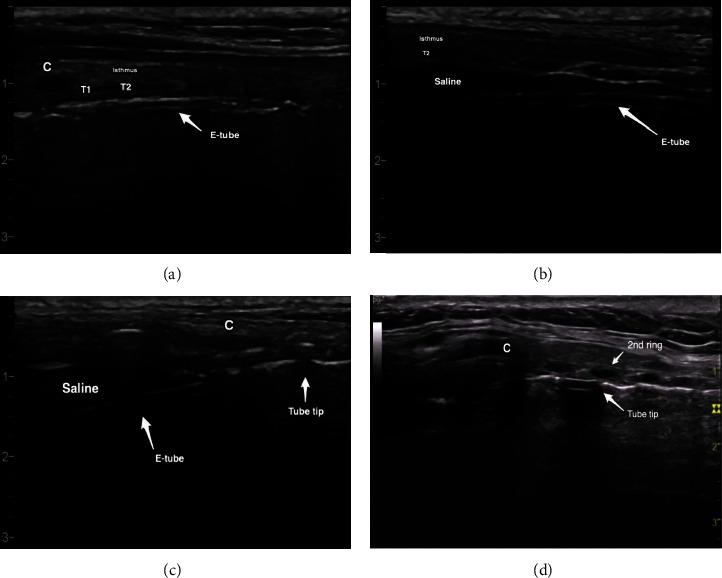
Endotracheal tube repositioning for percutaneous dilatational tracheostomy under ultrasound guidance. (a) Double-linear hyperechogenecity revealed the ET tube. (b) Anechoic filling and the ET tube were identified beneath the tracheal cartilage after saline ballooning. (c) Saline ballooning superior to the cricoid cartilage and pushing of the tracheal cartilage anteriorly by the ET tube tip were identified after pull back. (d) The ET tube tip was identified just superior to the 2nd tracheal ring in another patient. C: cricoid cartilage, ET tube: endotracheal tube, *T1 and T2*: 1^st^ and 2^nd^ tracheal ring, respectively.
